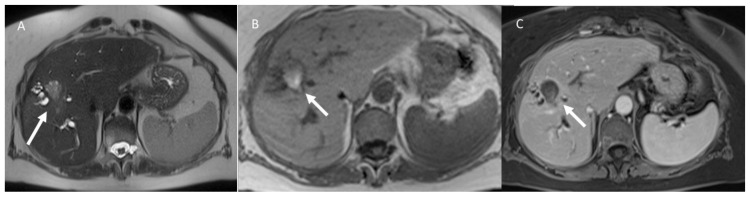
Woman 58 years at 1-month follow-up after microwave ablation of liver metastasis. MRI assessment (A) half-Fourier acquisition single-shot turbo-spin-echo (HASTE) T2-weighted sequence; in phase T1-weigthed sequence pre (B,C) post contrast assessment: ablated zone with biliary tree damage (arrow).
